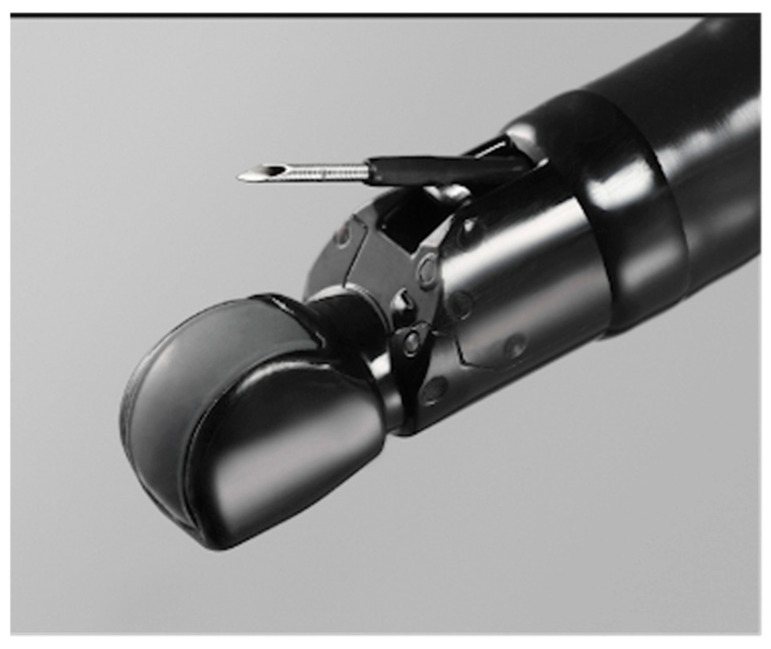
The main features of endoscopic ultrasound Fujifilm EG-740UT curved linear with optimized material elasticity to facilitate advanced force transmission, a short bending radius to facilitate access precision of the targeted area, optimized forward-viewing visualization and Fujifilm G-Lock elevator locking mechanism (19).
